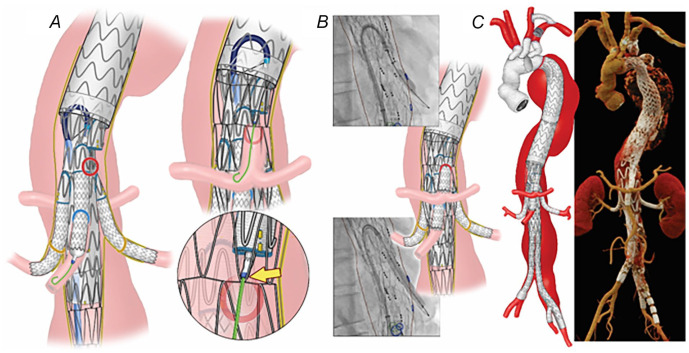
(A) Endovascular repair was performed using off-the-shelf multi-branched stent-graft through a total trans-femoral approach using a steerable sheath. Following sequential catheterization and stenting of both renal arteries and superior mesenteric artery, advancement of the stent into the celiac axis was not possible due to encroachment of the tip of the stent delivery system into one of the bare-metal stent struts. (B) A 6 Fr Shuttle sheath was introduced through the bare-metal stent struts and a short 6 × 22 mm iCAST balloon-expandable covered stent was placed. Subsequently, the celiac axis bridging balloon-expandable covered stent was successfully advanced and deployed without difficulty. (C) Postoperative 3-dimensional computed tomography angiography reconstruction shows widely patent branched stent-graft.
